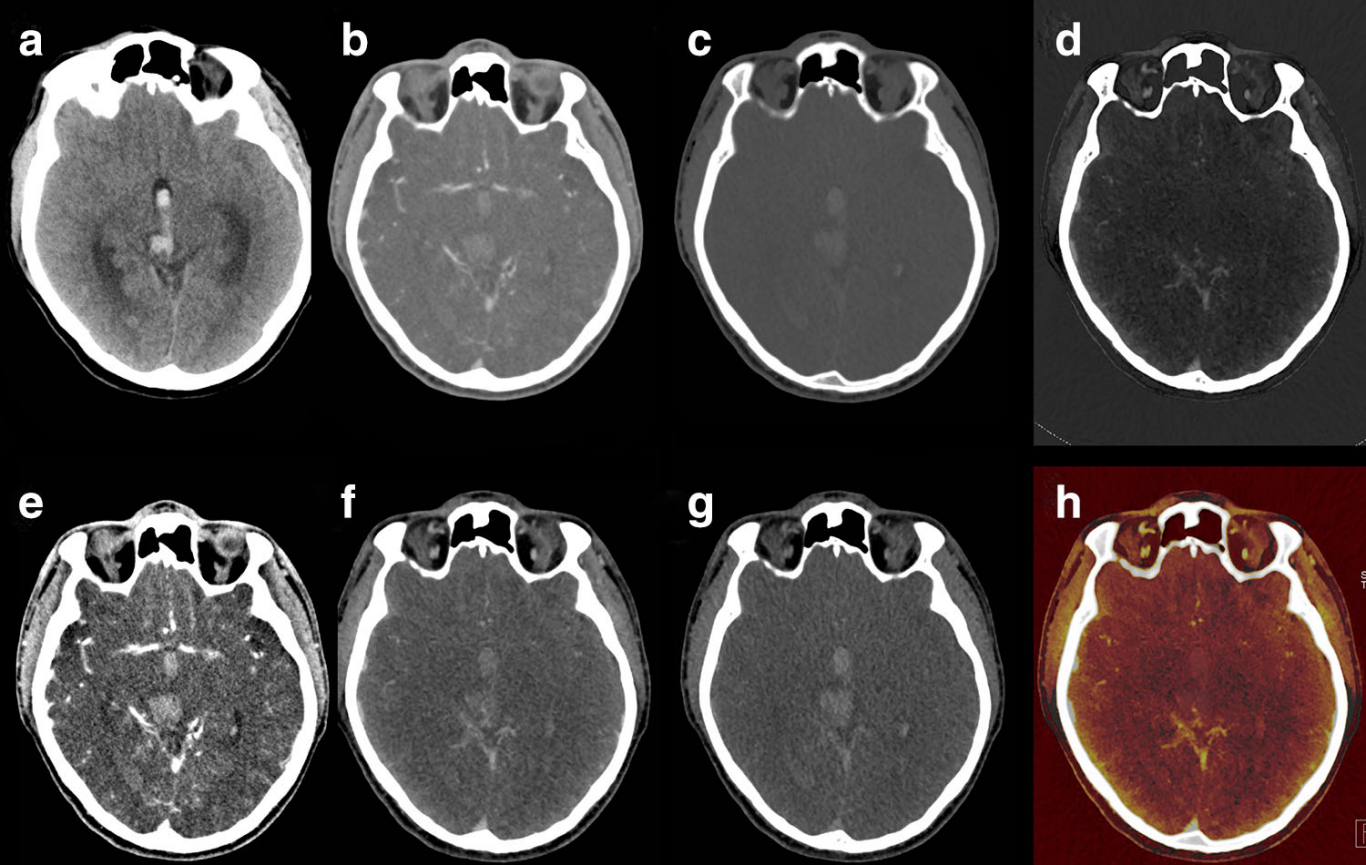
55-year-old male presented with midbrain hemorrhage. DECTA was subsequently performed. Shown are NECT (*a*), mixed images DECTA H20 kernel (*b*), VNC (*c*), IOM (*d*), mixed images DECTA H31 kernel (*e*), 80 kVp images (*f*), 140 kVp images (*g*) and fusion images (*h*). NECT at presentation showed a small mesencephalic hemorrhage. DECTA mixed images at H20 (*b*) and H31 (*e*) kernels did not show any underlying abnormalities. However, a small vascular malformation was appreciated at the IOM (*d*), 80 kVp (*f*) and fusion images (*h*), clearly demonstrating the additive value of DECT reconstructions (conventional CT 120 kVp, 285 mAs, CTDI 48.28 mGy, DLP 833 mGycm. DECTA 80/Sn140 kVp, 310/155 mAs, CTDI 26.34 mGy, DLP 475 mGycm; 95 cc iodinated contrast 300 mg/ml; injection rate 5.5 cc/s; 40 cc saline flush 5.5 cc/s)
